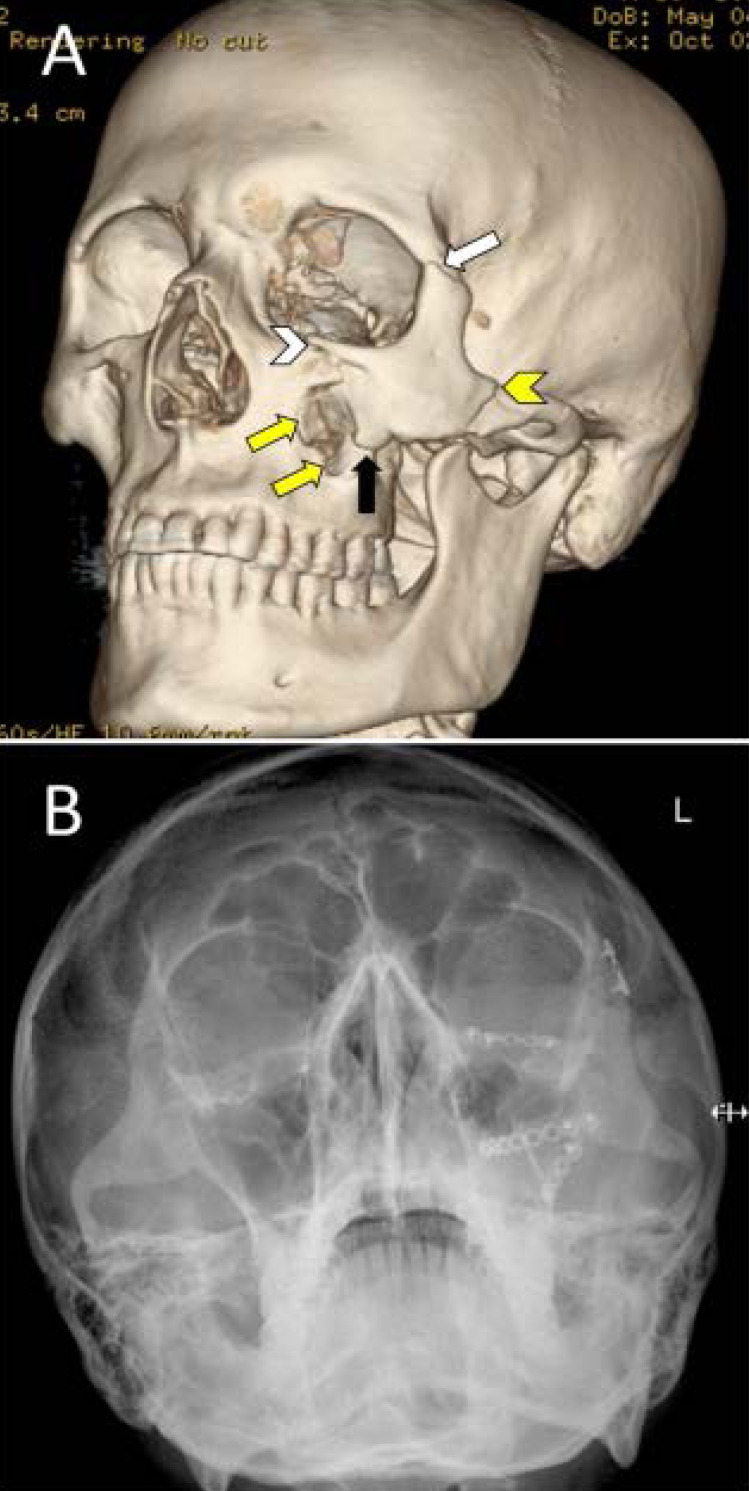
A 30- year old camel caregiver fell off while riding a camel followed by being stepped on his left side of the face by another camel. A three-dimensional (3D) CT scan (A) demonstrates ZMC fracture with disruption of the left zygomaticofrontal suture (white arrow), the zygomaticomaxillary suture (black arrow), and the zygomaticotemporal suture (yellow arrowhead). Note also the displaced fracture of the left orbital floor (white arrowhead) and the anterior wall of the maxillary sinus (yellow arrows). Postoperative plain radiograph (B) showing reconstructed facial fractures with open reduction and internal fixation.
